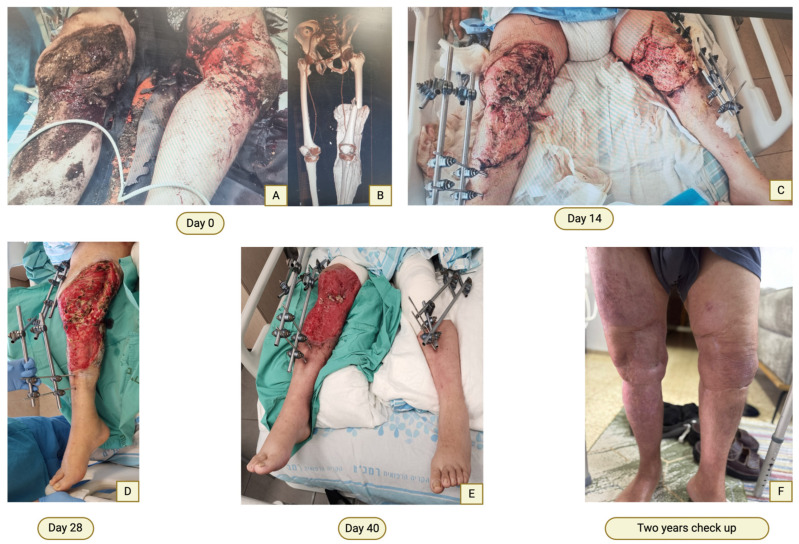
Progressive debridement and wound bed reactivation following COD therapy in a contaminated open fracture. (A) Day 0: extensive soft tissue devitalization following high-energy open fracture with severe contamination. (B) CT angiography demonstrates skeletal injury with preserved arterial perfusion. (C) Day 14: persistent devitalized tissue despite prior surgical debridement and antiseptic wound care. (D) Day 28: marked replacement of necrotic tissue by dense granulation tissue following COD therapy. (E) Day 40: well-vascularized wound bed suitable for skin grafting. (F) Long-term follow-up demonstrating stable wound closure and limb preservation.
